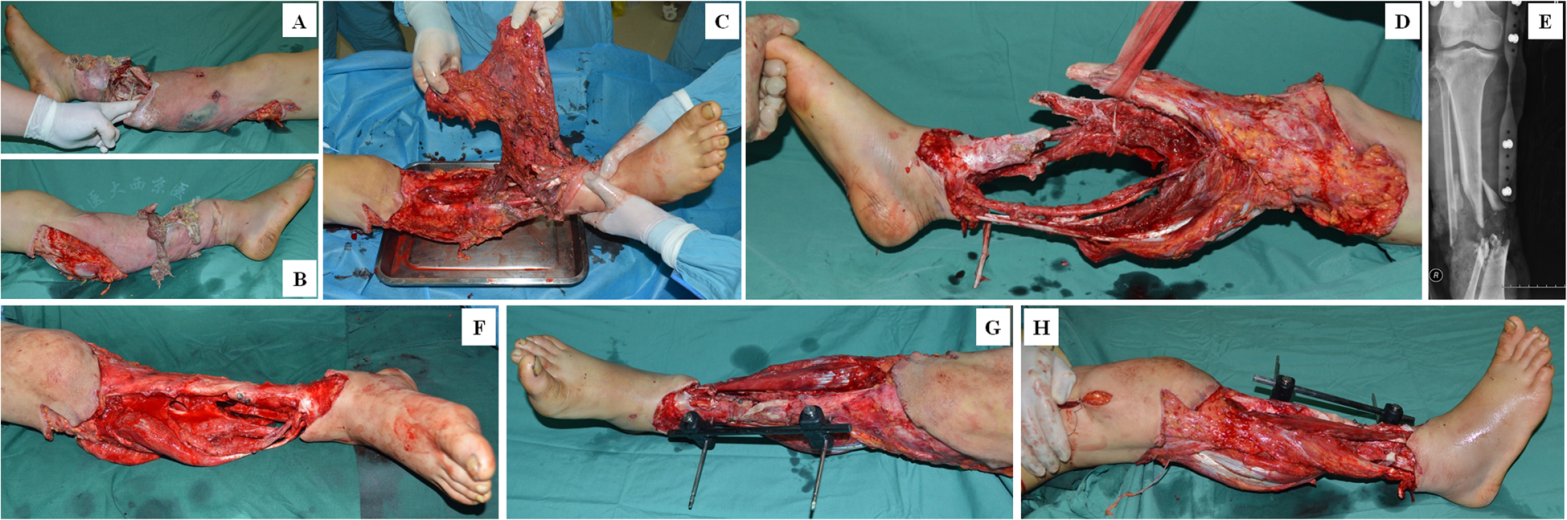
Case 1-1. Appearance of right leg due to a blunt injury. (A-C). Appearance of right leg after debridement (D). Radiographic examination of right tibial and fibular fractures (E). Appearance of right leg after shortening and external fixation (F-H)
